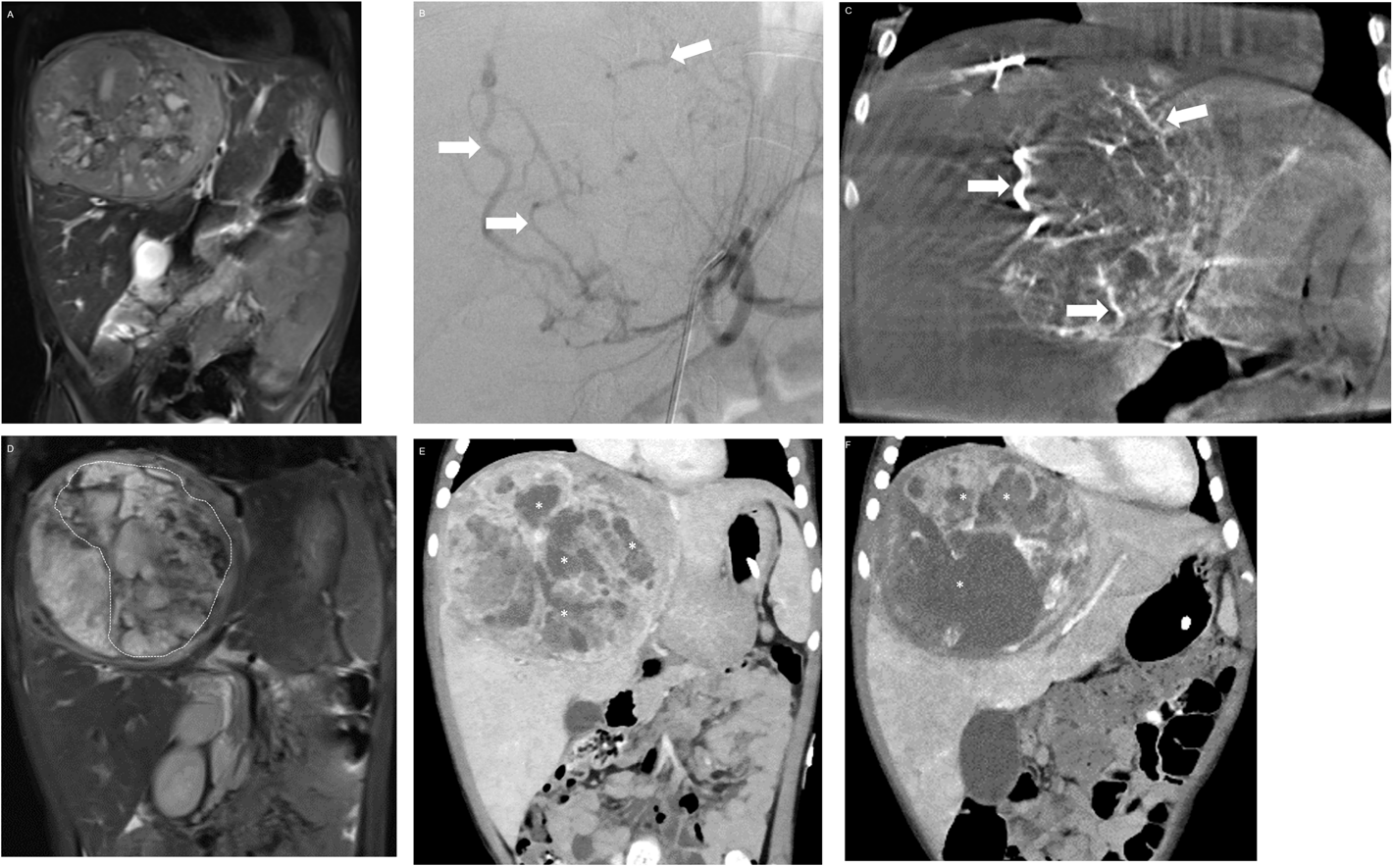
T2- weighted fat saturated coronal (a) MR image demonstrating a large hepatocellular carcinoma in a 2-year-old male patient which was unresponsive to systemic chemotherapy. b Angiography obtained during transcatheter arterial chemoembolization demonstrating several hepatic arterial branches encasing and supplying the tumor (arrows). These feeders were injected with 30 mg of doxorubicin emulsified with lipiodol followed by embolization with 150–250-μm poly vinyl alcohol particles confirmed on cone-beam computed tomography (c) obtained post embolization demonstrating the radio-opaque embolization beads in the tumor bed (arrows). d Post embolization T1- weighted post contrast coronal MRI obtained 3 weeks later demonstrating heterogeneous necrotic area (dotted line) within the tumor. Coronal computed tomography obtained 3 weeks (e) after the first TACE procedure and 3 weeks (f) after the second TACE procedure demonstrating increasing necrotic areas within the tumor (asterisk). The patient underwent a successful liver transplant 6 weeks later
